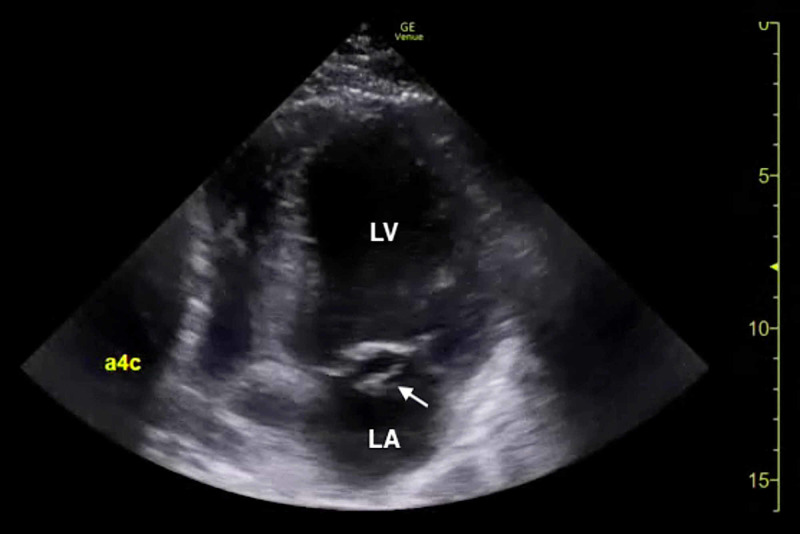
Bedside transthoracic echocardiogram (apical window) demonstrating a flail posterior mitral leaflet (arrow). LA, left atrium; LV, left ventricle.
